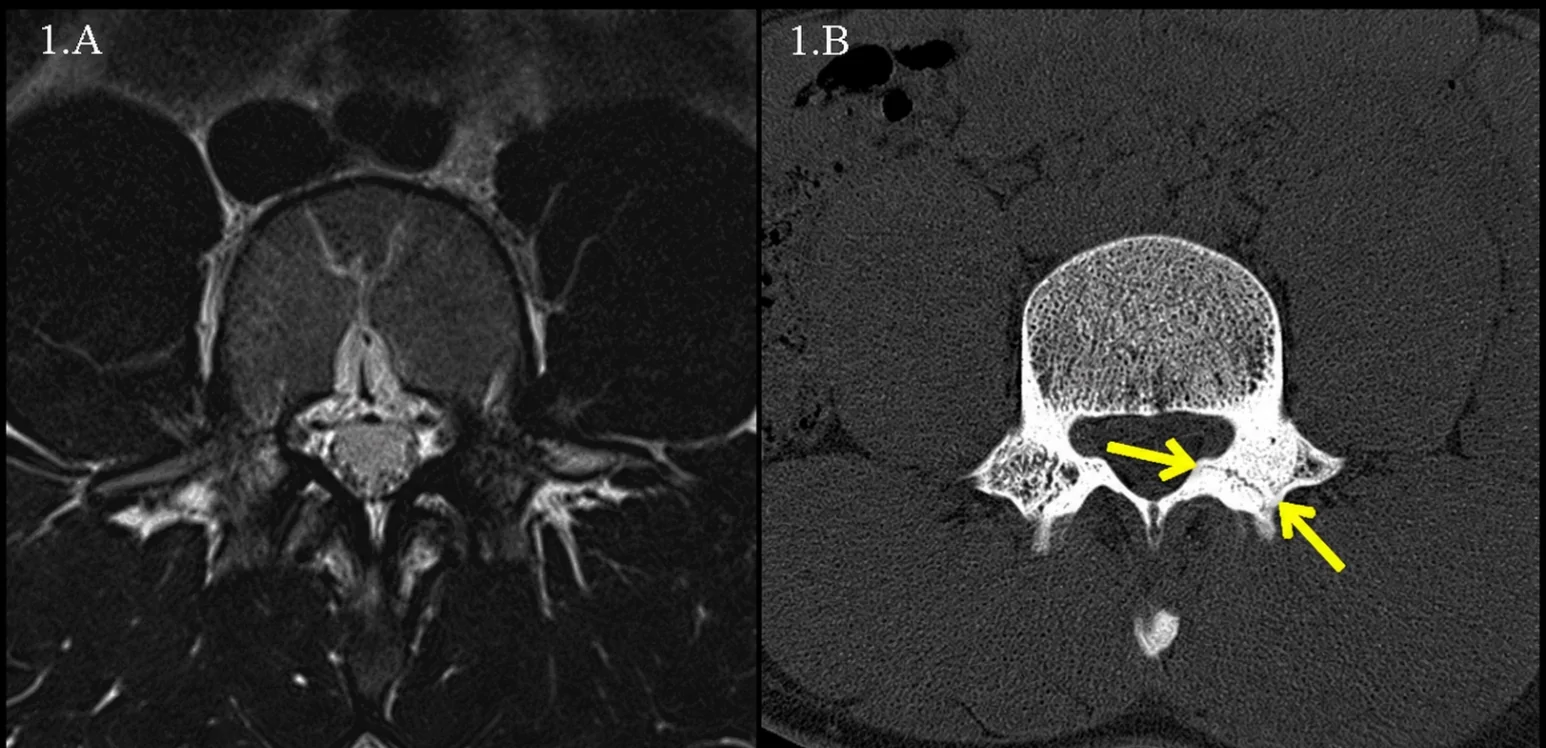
A: Axial T2 MRI sequence of L4 vertebra; B: Axial CT sequence of L4 vertebra. Yellow arrow indicates stress fracture. CT, computed tomography; L4, fourth lumbar vertebra; MRI, magnetic resonance imaging.
